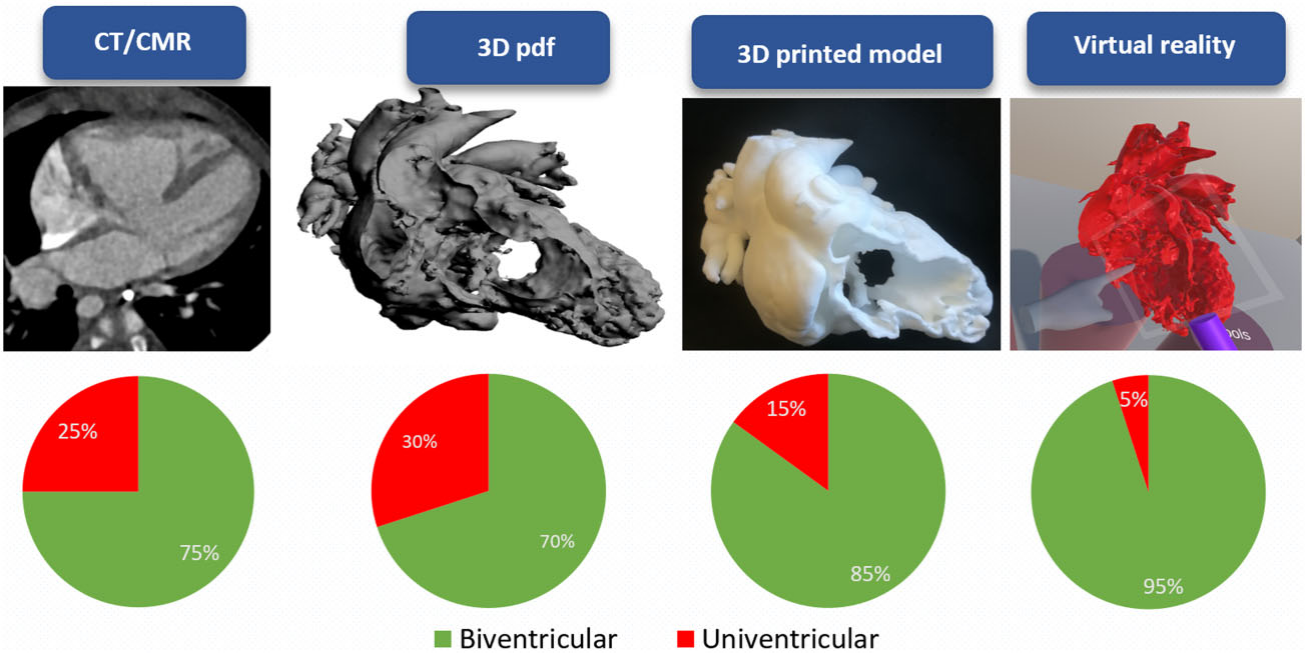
Visual summary of the level of accordance (%) between the two surgeon evaluators and the actual performed plan in relation to the imaging modality used. CMR, cardiac magnetic resonance; CT, computed tomography; 3D, three-dimensional.
